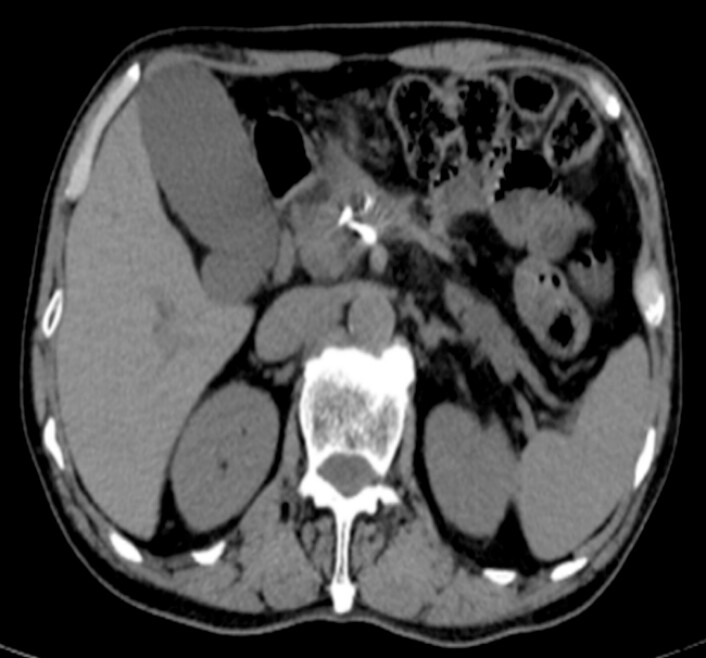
CT imaging demonstrates a significant reduction in the fistula cavity within the body of the pancreas, with no significant dilation observed in the main PD in the tail of the pancreas. CT, computed tomography; PD, pancreatic duct drainage.
